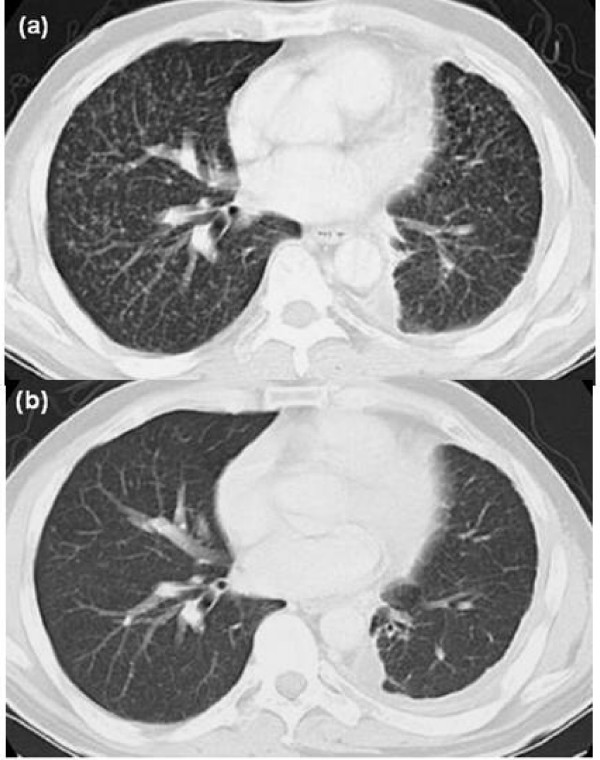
Radiological evaluation of response to initial treatment with gefitinib on CT cans. (a) before treatment, (b) after treatment.
